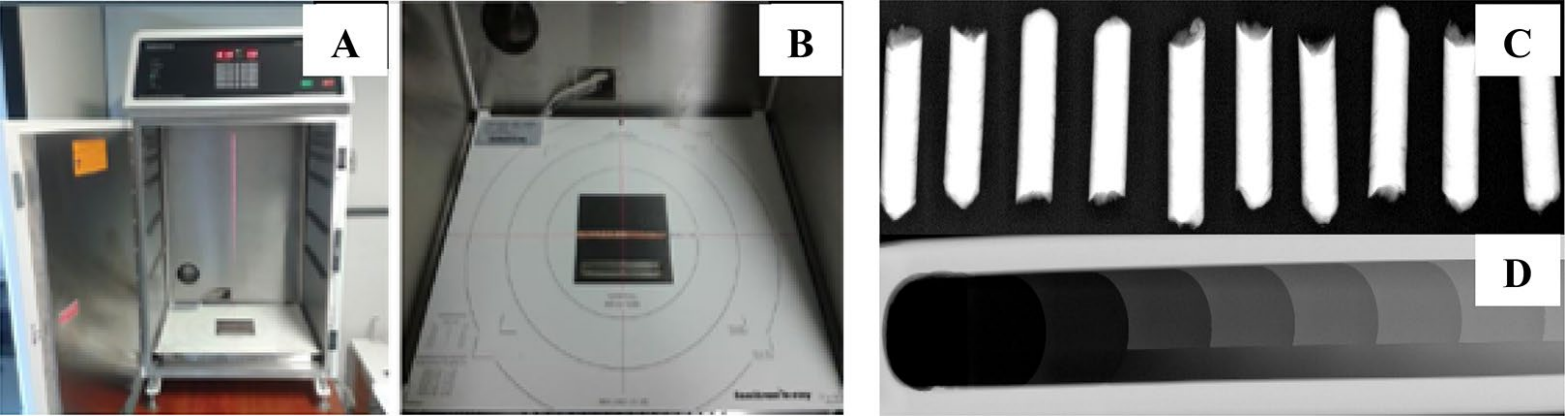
X-ray densitometry. (A) Faxitron model LX-60 cabinet X-ray system. (B) Shielded X-ray compartment. (C) Pellets. (D) Cellulose acetate calibration wedge.
